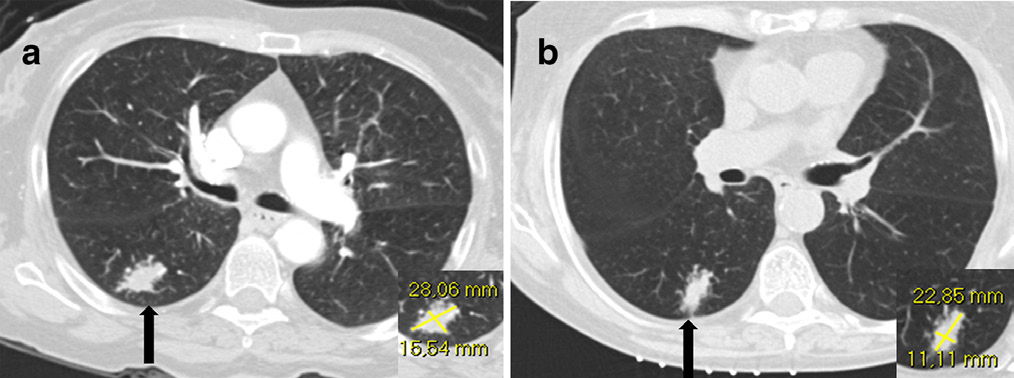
A 60-year-old female was admitted to the hospital because of headache (patient no. 11 in Table 1). CT and MRI of the brain (not shown) demonstrated multiple cerebellar lesions suspicious for metastases. Subsequently, a CT scan of the thorax and abdomen was performed which revealed a lesion in the lung (1A) (black arrow) of 28 mm long-axis diameter. On prebiopsy planning CT, this lesion had decreased in size to 23 mm (1B) (black arrow). The attending radiologist decided to proceed with biopsy. Subsequent pathological examination revealed a large-cell neuroendocrine carcinoma.
